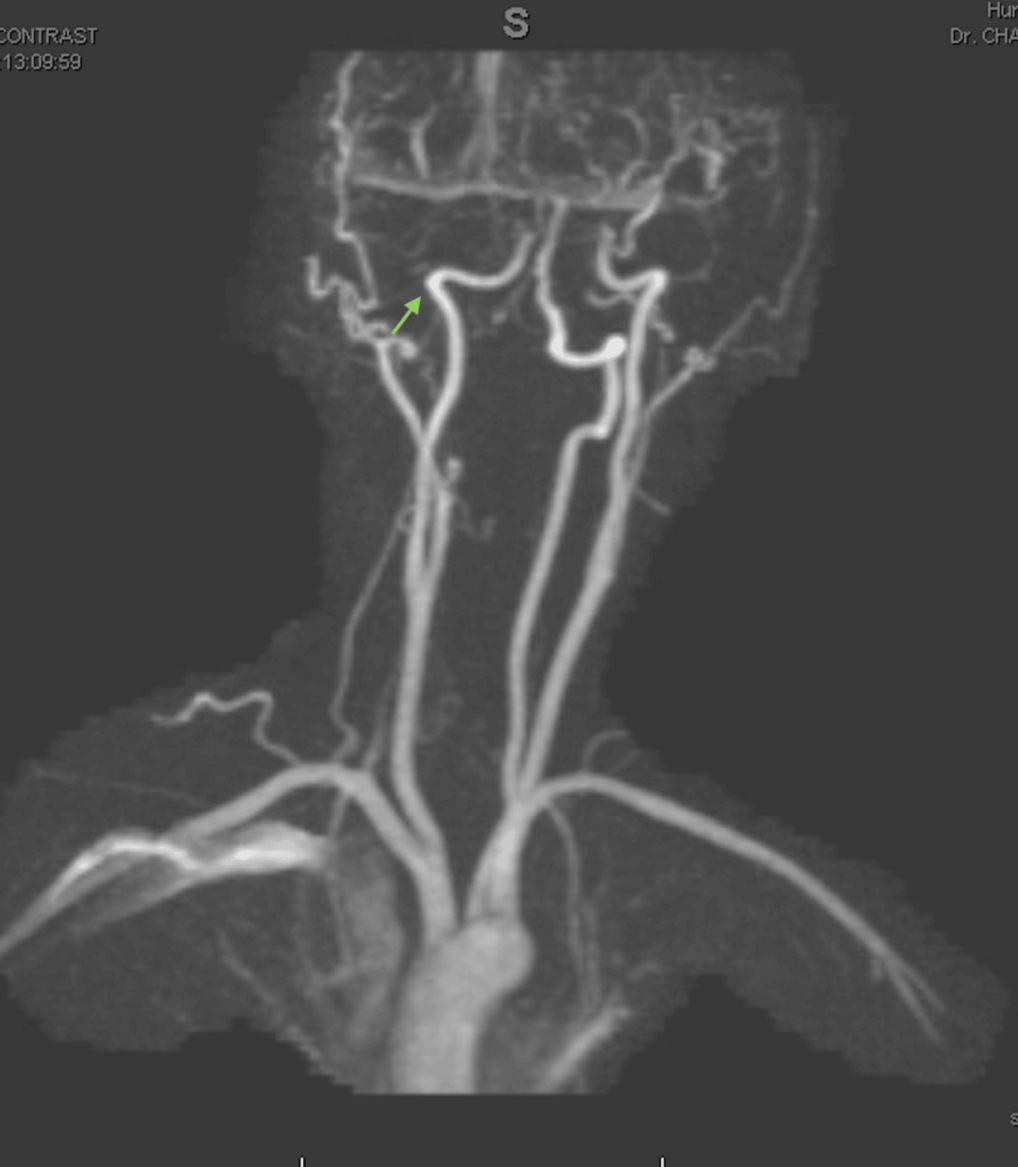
MRI of the neck showed occlusive dissection V3 segment of the right vertebral artery and reconstitution of minimal flow along the V4 segment, which may be partially attributed to retrograde flow.
